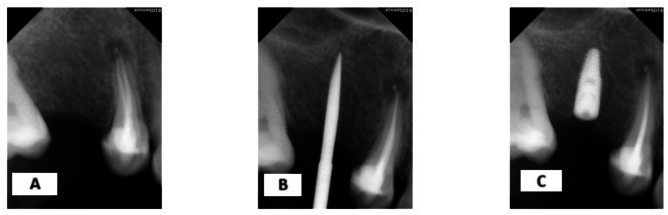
Preoperative, intraoperative, and postoperative periapical radiographs showing implant site status and placement accuracy.
